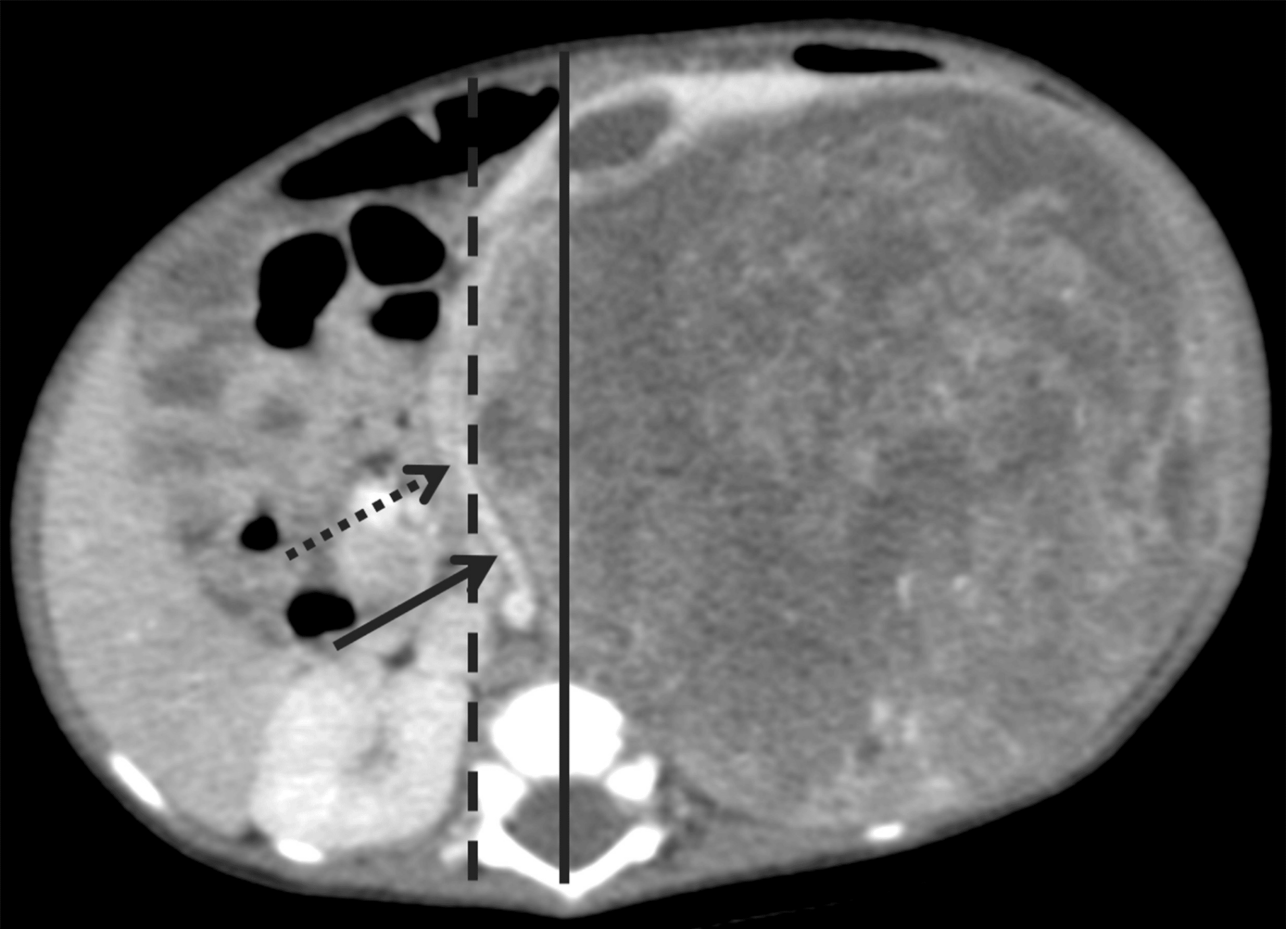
The axial CT image shows the definition of contralateral tumor extension (dashed line), left renal pedicle (dashed arrow), and left renal artery (straight arrow) involvement (midline of the vertebral body = straight line). CT: computed tomography
